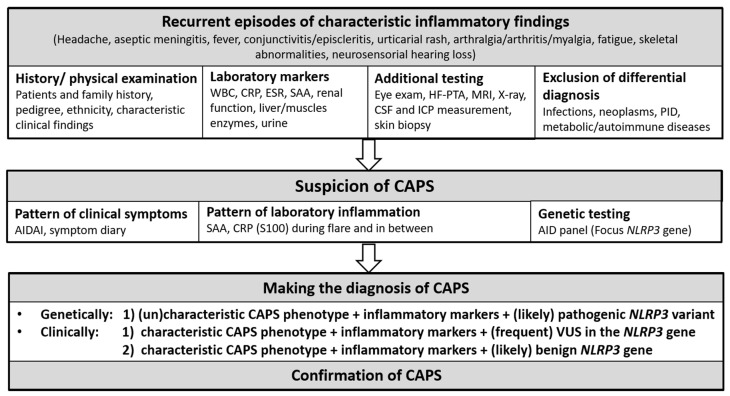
Diagnostic approach to CAPS. WBC, whole blood count; CRP, c reactive protein; ESR, erythrocyte sedimentation rate; SAA, serum amyloid A; HF-PTA, high frequency pure tone audiogram; MRI, magnet resonance imaging; CSF, cerebrospinal fluid; ICP, intracranial pressure; PID, primary immune deficiency; AIDAI, autoinflammatory disease activity index; S100, S 100 proteins (S100A12, S100A8/A9); VUS, variant of uncertain significance. Bold format and grey background indicate headings.
